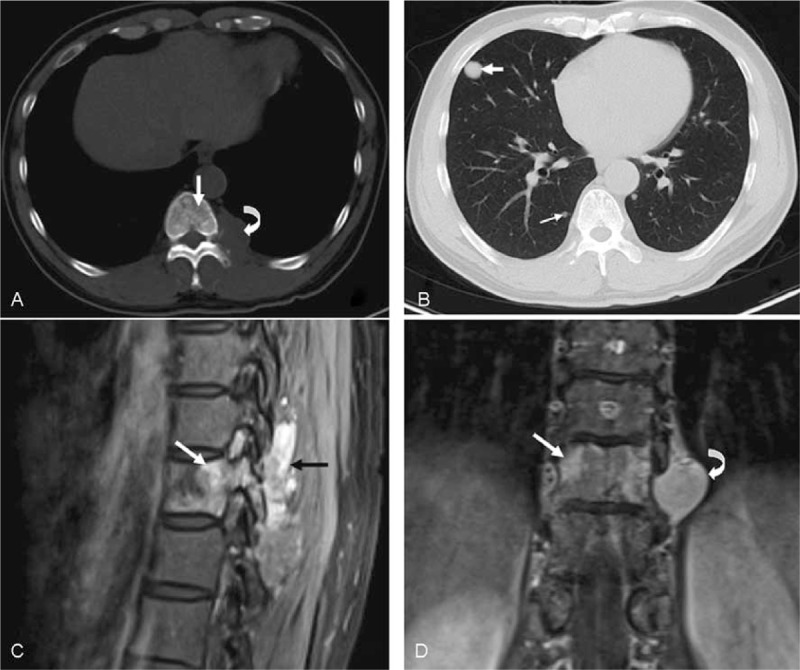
Typical features of malignant SFTs. A mass was found that originated from the pleura and was metastasized to the lung. CT mediastinal windows (A) showed a homogeneous lesion (curved arrow) in the left pleura invading to the neighboring T10 vertebral body (arrow, confirmed pathology). Parenchymal windows (B) showed metastases (arrows) in the right lung. Lesions (arrows) are marked and heterogeneously enhanced on T1-weighted MRI scans (C and D). CT = computed tomography, MRI = magnetic resonance imaging, SFTs = solitary fibrous tumors.
